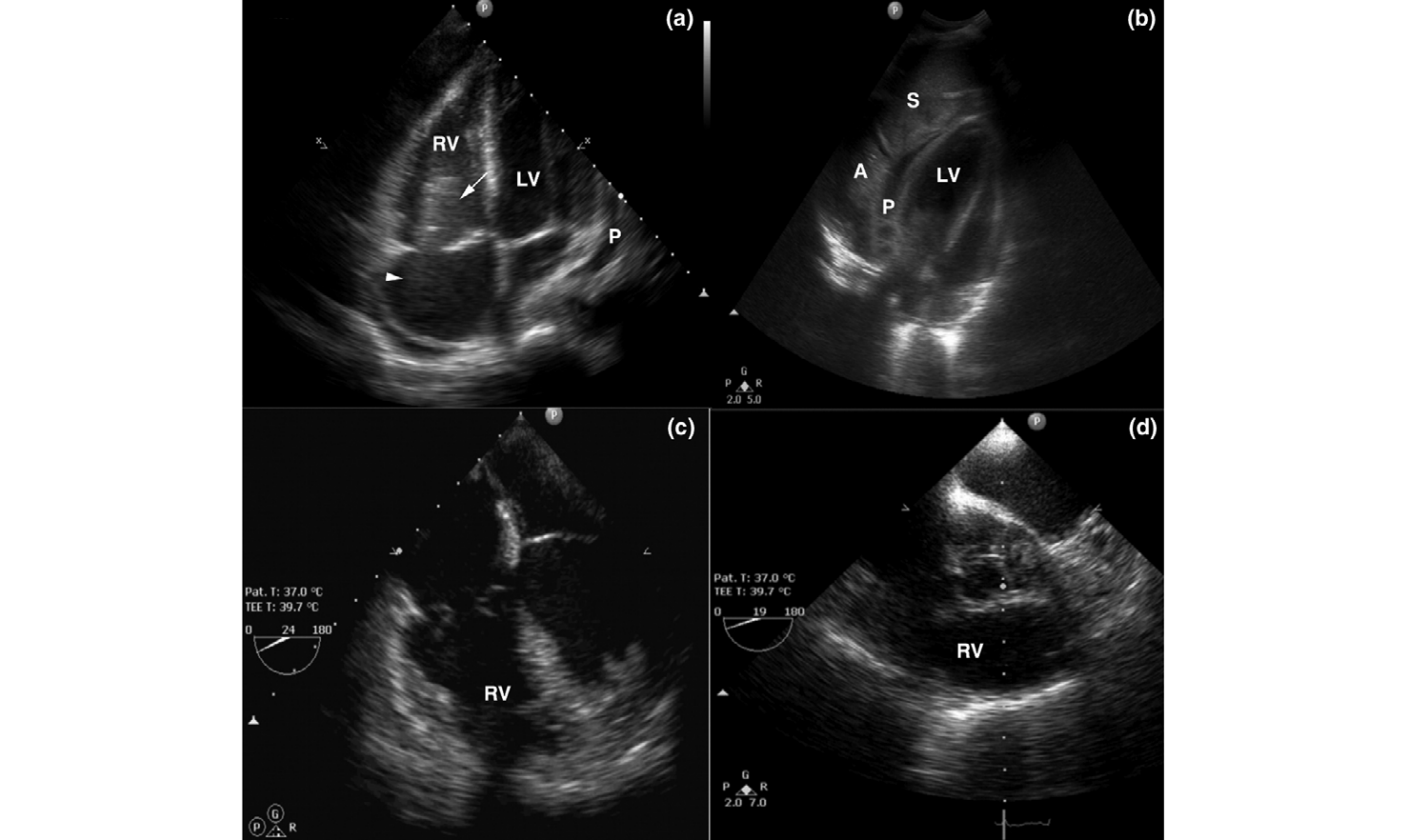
Chest echography (a,b) and transesophageal echocardiography (c,d) (LV, left ventricle; P, pericardial fluid; S, spleen). (a) Apical four-chamber view of the artifact in the right ventricle; (b) echography demonstrating the lung atelectasis, the spleen and the pericardial fluid in the same patient; and (c,d) transesophageal echocardiography, revealing no abnormalities.
